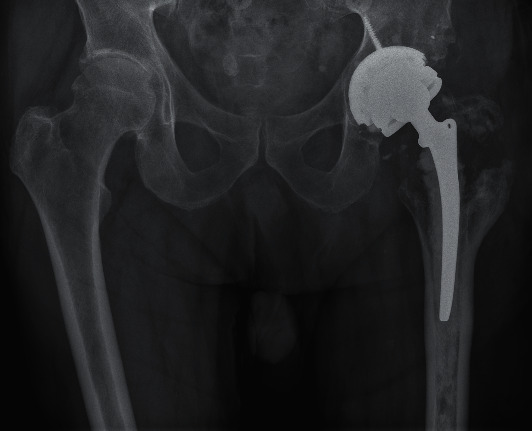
X-ray after the third revision surgery (2020).
